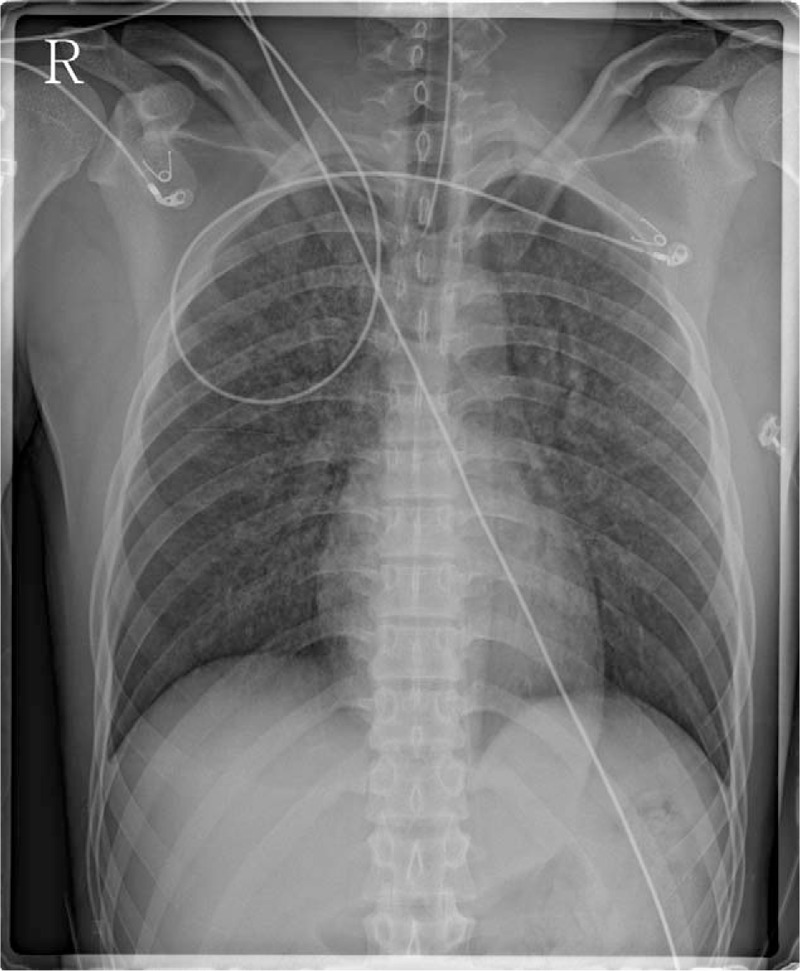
Portable chest anteroposterior radiograph obtained 17 minutes after re-intubation showing diffuse alveolar infiltration in both lungs.
